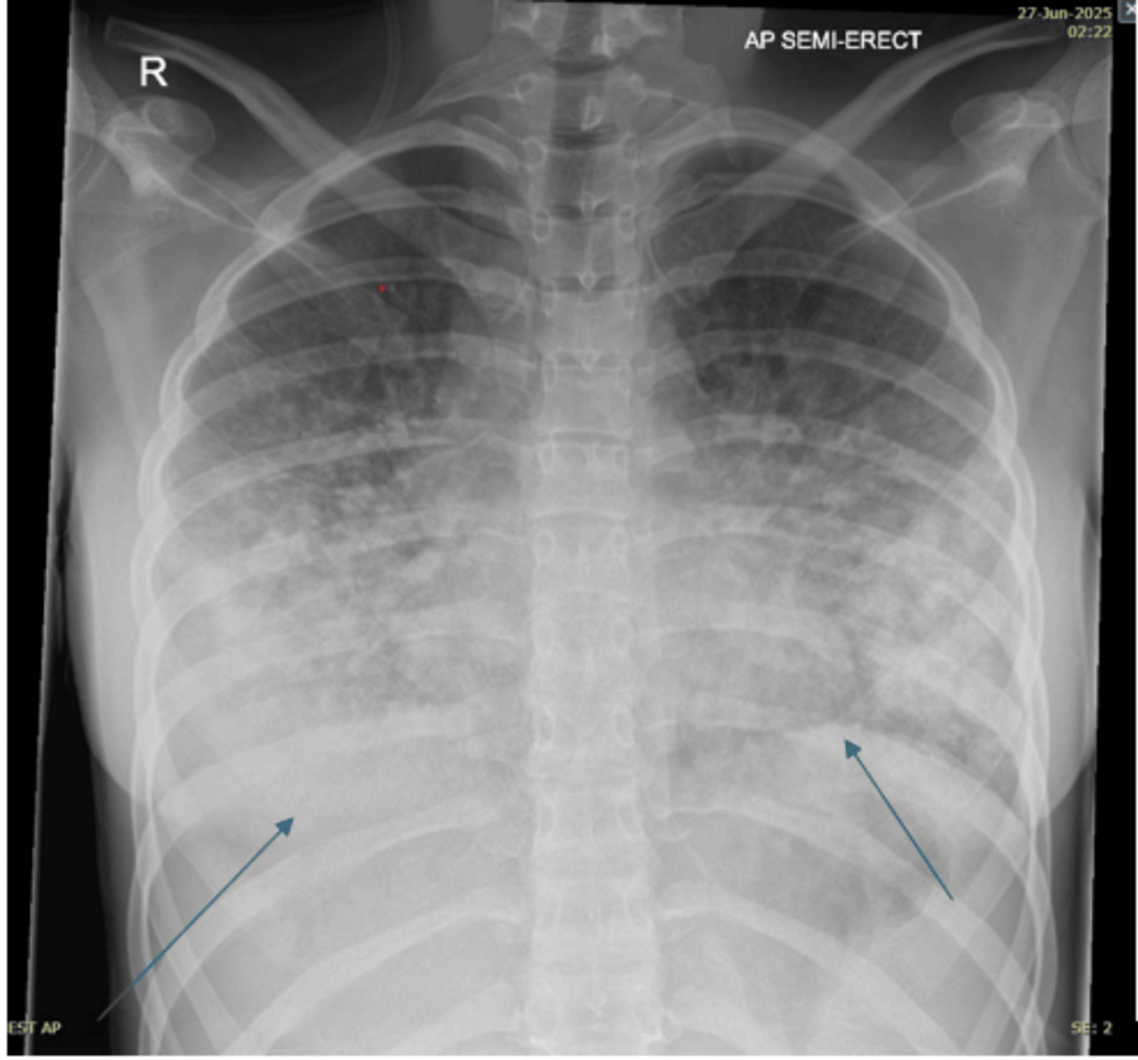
Chest X-ray of our patient showing inflammatory changes.
